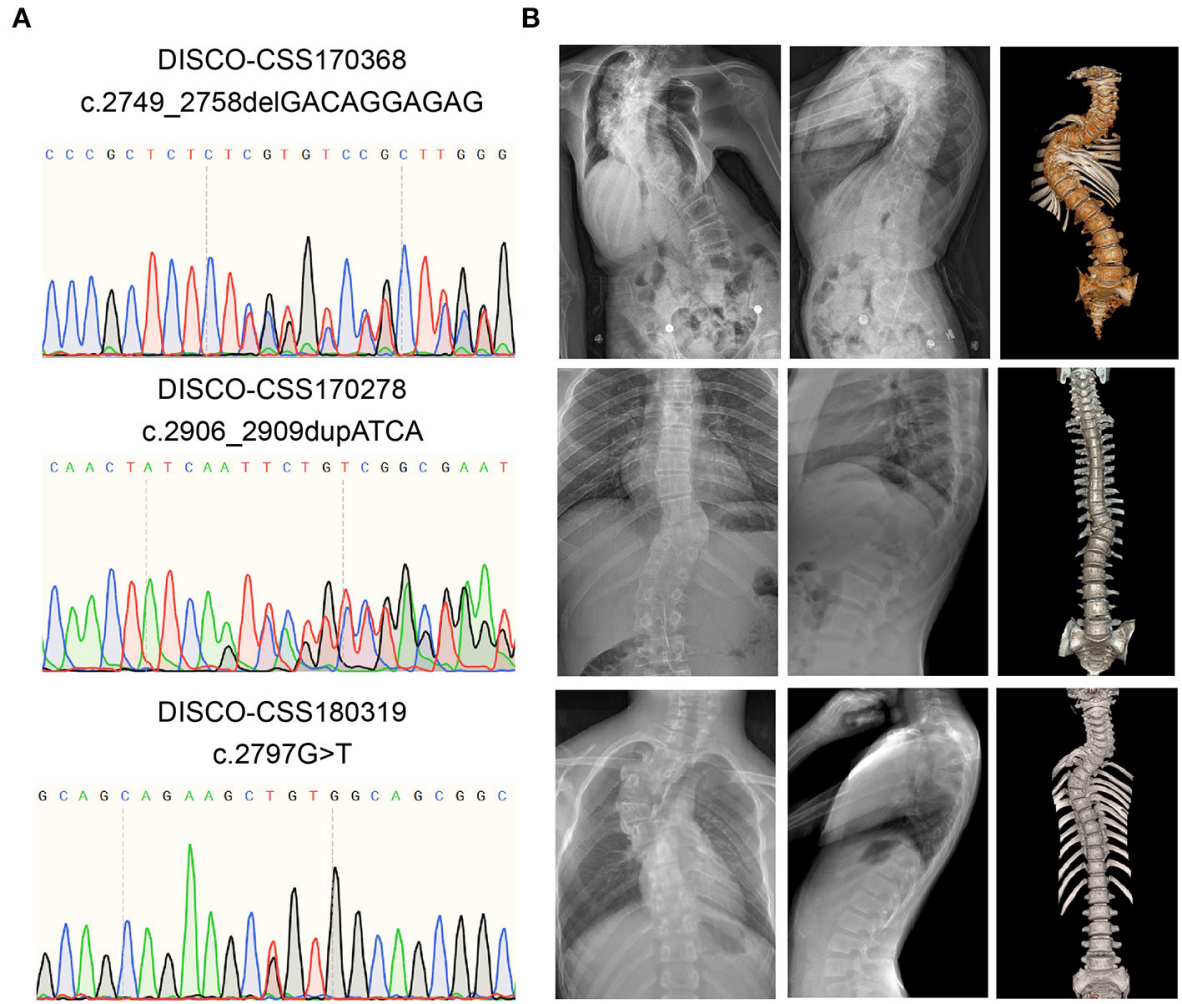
Variant information and clinical phenotype of *CSF1R* carboxy-terminal variants in three patients with CVM. (A) Results of Sanger sequencing of the rare *CSF1R* carboxy-terminal variants. (B) Spinal plain radiograph and computed tomography (CT) scan three-dimensional reconstruction of those CVM patients. CVM, congenital vertebral malformation.
